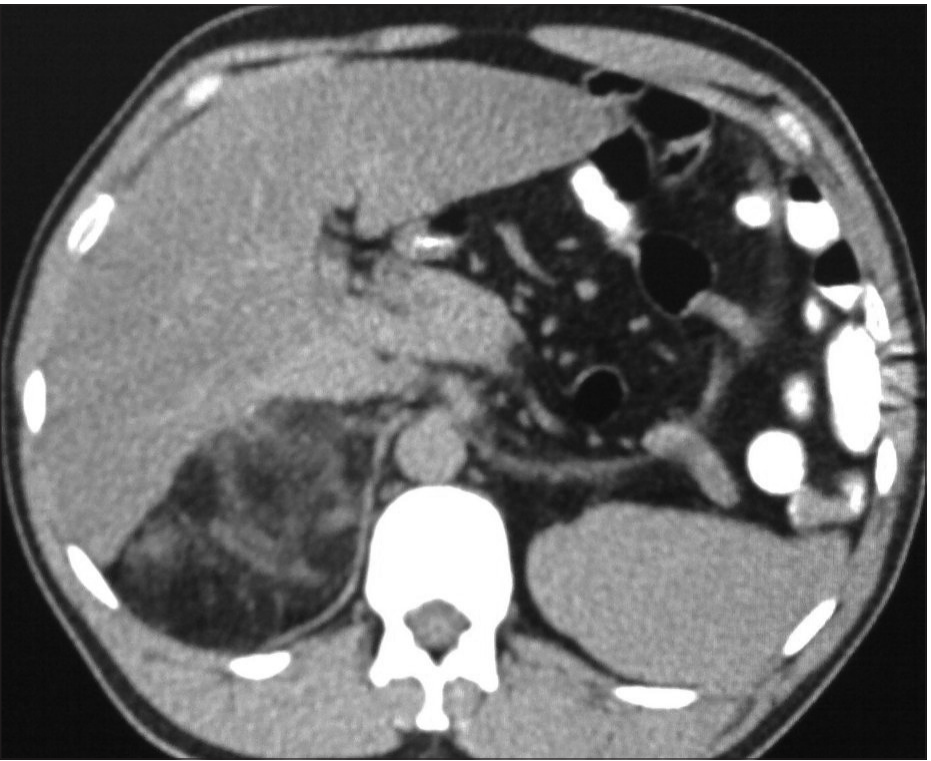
Noncontrast enhanced transverse CT image showing a large fat density mass in the right adrenal with some denser components in it; left hemidiaphragm shows posterolateral defect with luminal contrast filled stomach and small intestine and spleen posterior to it inside left hemithorax
